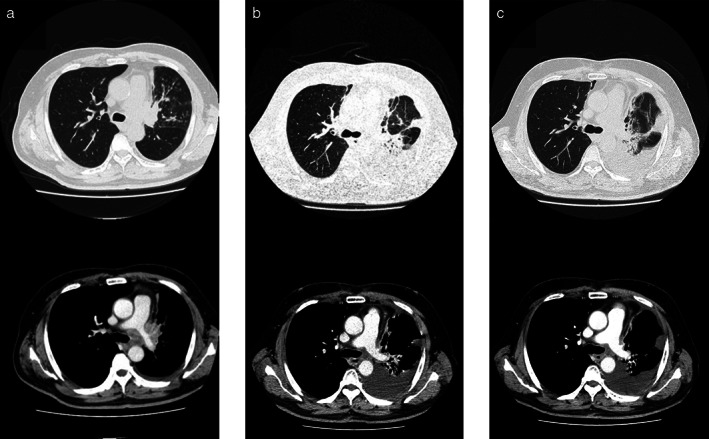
Computed tomography (CT) findings. (a) Before chemoradiation therapy, a mass was detected in the left lung hilum. (b) CT during atezolizumab treatment revealed shrinking of the left hilar mass. (c) CT of hemoptysis with no exacerbation of the left hilar tumor
